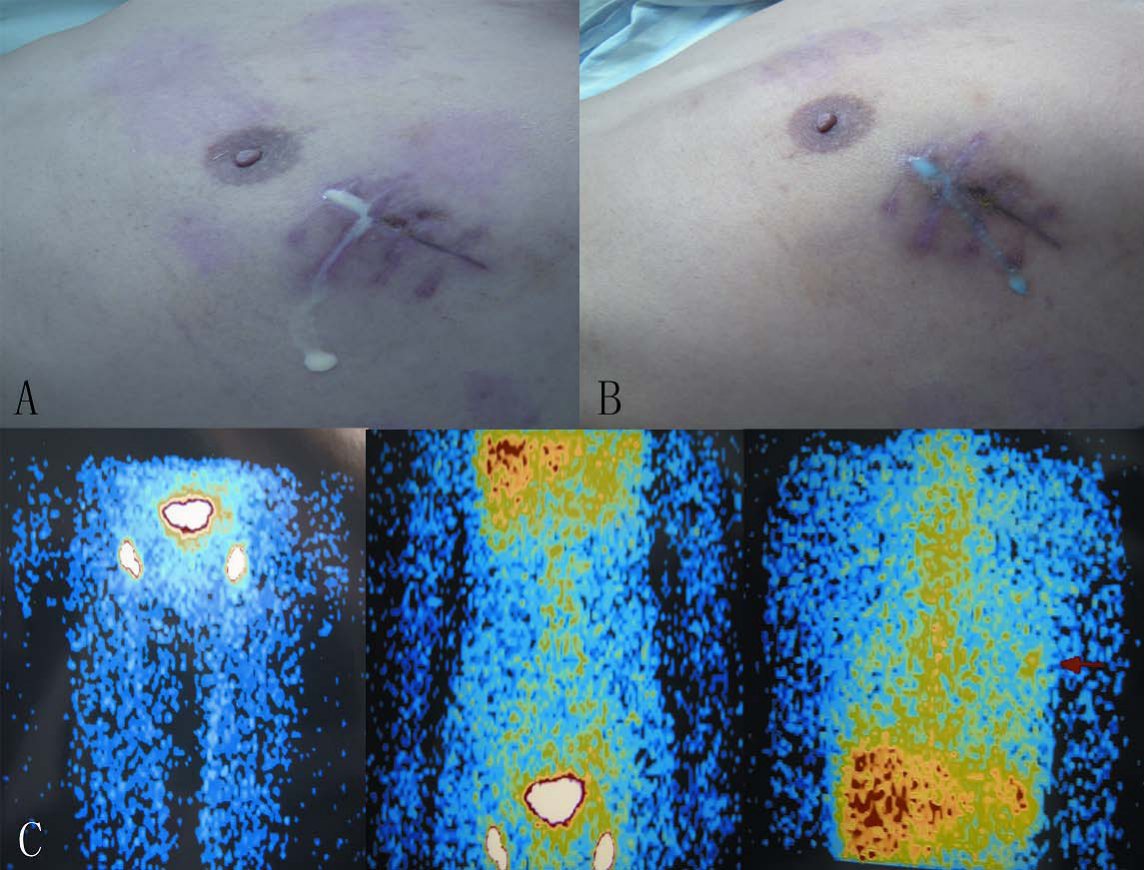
Chyle leakage in port incision in the left chest wall and its lymphoscintigraphy. A: Milky white and odorless liquid leaked from the front of the major port incision. B: The leakage became blue after the patient ingested Sudan Black. C: Lymphoscintigraphy using Tc-99 m sodium phytate as a tracer. The image was taken 60 min after injection. An abnormal tracer accumulation was evident in the left chest wall corresponding to the major incision (red arrow). No tracer accumulation was found in the pleural cavity.
